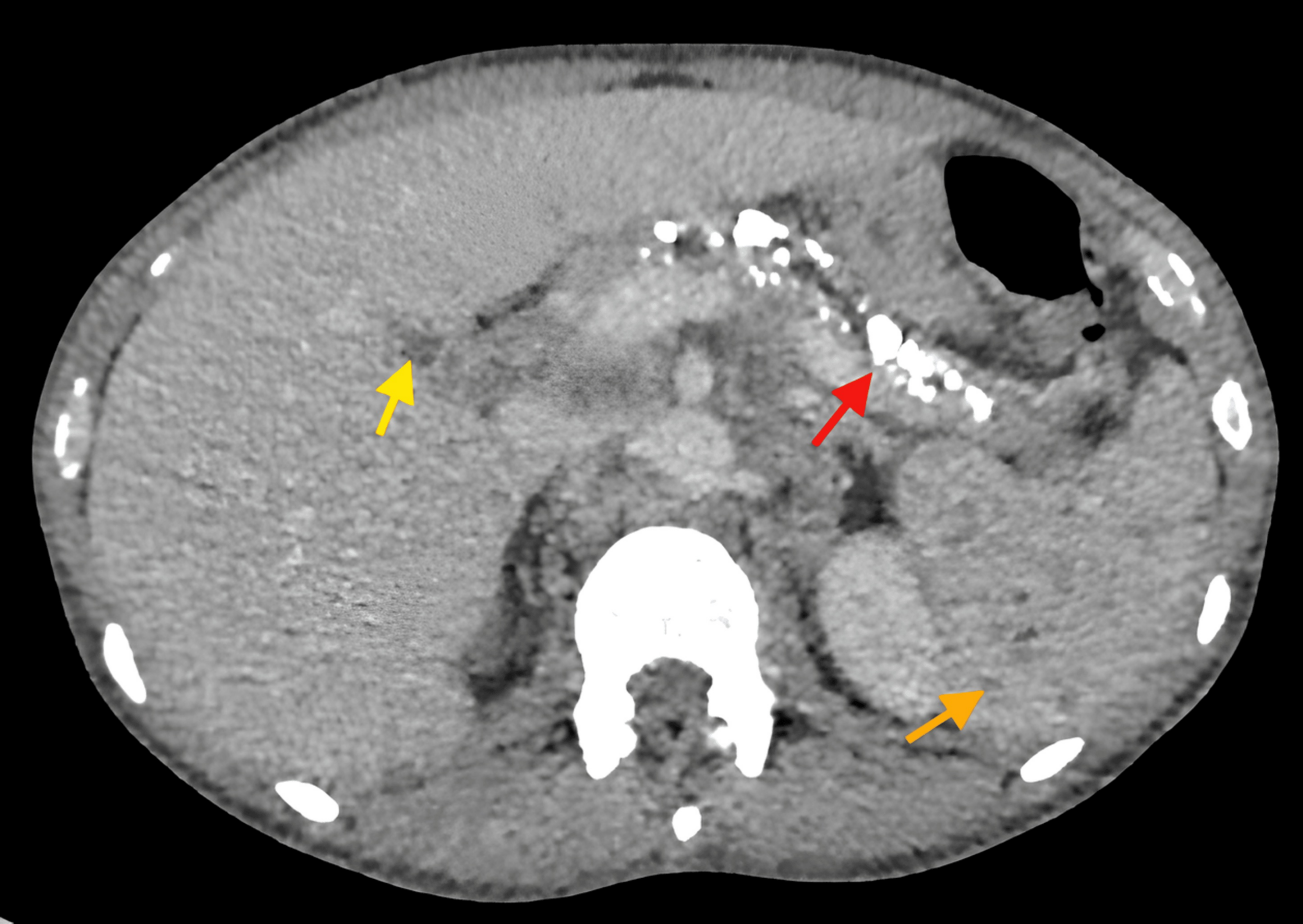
CT of the abdomen (axial view) showing multiple tiny hypoenhancing splenic nodules, extensive pancreatic calcification and one hypoenhancing liver nodule Golden arrow: spleen, red arrow: pancreas, yellow arrow: liver CT: computed tomography
